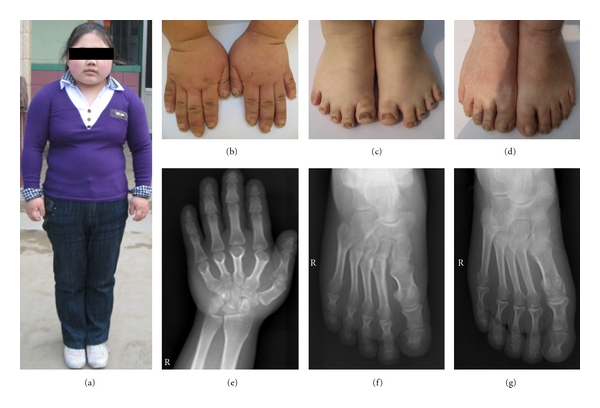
(a) The proband appeared short, obese, and face round. (b) The proband's hands. (c) The proband's feet. (d) Feet of the proband's mother. (e) An X-ray of patient's right hand showing that her metacarpals and phalanges are generally shortened. The both ends of the twice-fifth metacarpals are enlarged. The first distal phalanx, twice-fifth middle phalanges and twice-fifth distal phalanges are thickened. (f) The X-ray of the patient's right foot showing that her metatarsus and phalanges are generally shortened, especially the first metatarsus and phalanx. The distal ends of the first metatarsus, first phalanx, and twice-fifth phalanges are enlarged. The proximal ends of the first phalanx and twice-fifth phalanges are widened. (g) The feet of the patient's mother looked normal; however, an X-ray of her right foot showed that she had a similar, but less severe, phenotype to her daughter.
